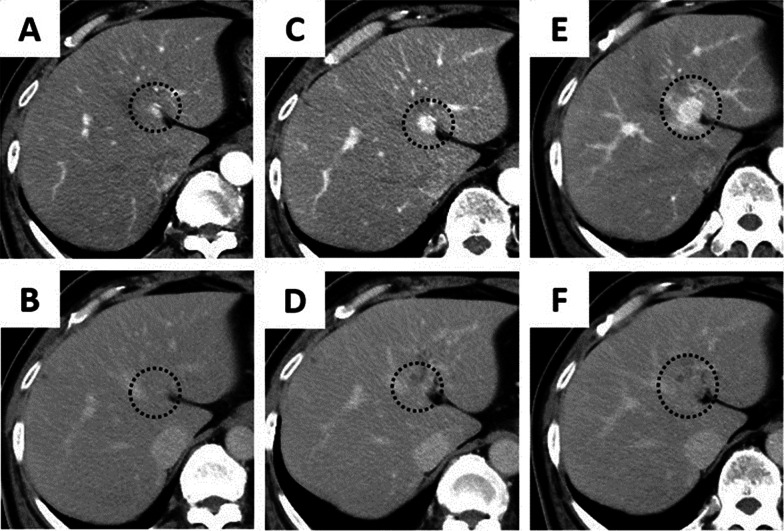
Dynamic abdominal computed tomography (CT) findings. A In 2015, the arterial phase showed enhancement of tumor at liver segment 4, measuring 10 mm in diameter. B The tumor was isodense during the late phase. C In 2017, the arterial phase showed enhancement of the tumor, measuring 15 mm in diameter. D The tumor remained isodense during the late phase. E In 2020, the arterial phase showed tumor progression, measuring 20 mm in diameter. The tumor was located close to the left first branch of Glisson. F The tumor remained isodense during the late phase
